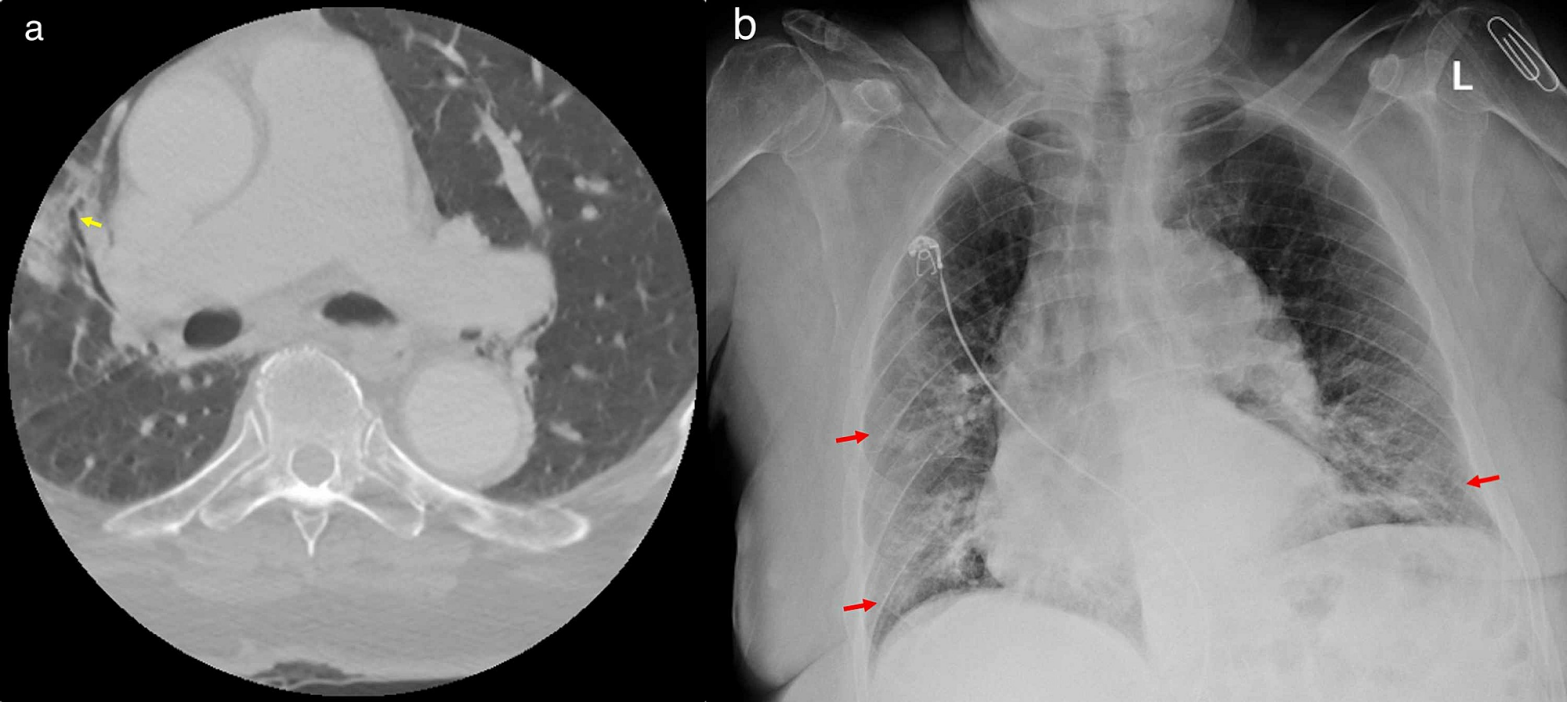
CT and chest radiograph of the patient (a) Axial image from a CT cervical, thoracic, and lumbar spine without contrast at the level of the mid lungs displays ground-glass infiltrates in the right middle lobe (yellow arrows). (b) Subsequent chest radiograph demonstrates bilateral lung infiltrates, asymmetric to the right (red arrows) CT: computed tomography
